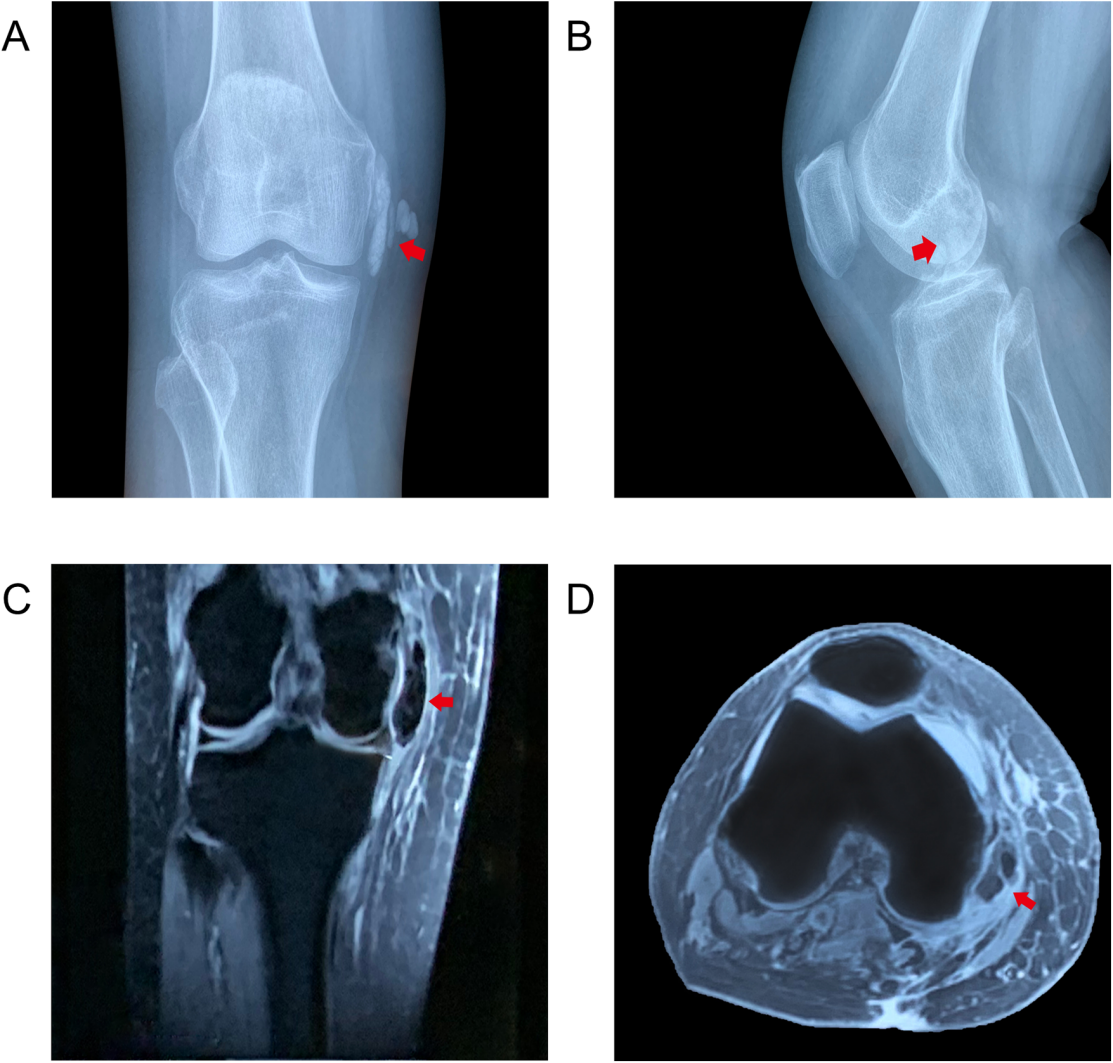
X-ray of right knee joint showed striped dense calcification in the MCL (red arrow): (A) coronal and (B) sagittal views. MRI showed the calcific mass showing low signal intensity, suggesting calcific deposition in the lateral side of the MCL: (C) T1 coronal and (D) T1 axial views.
